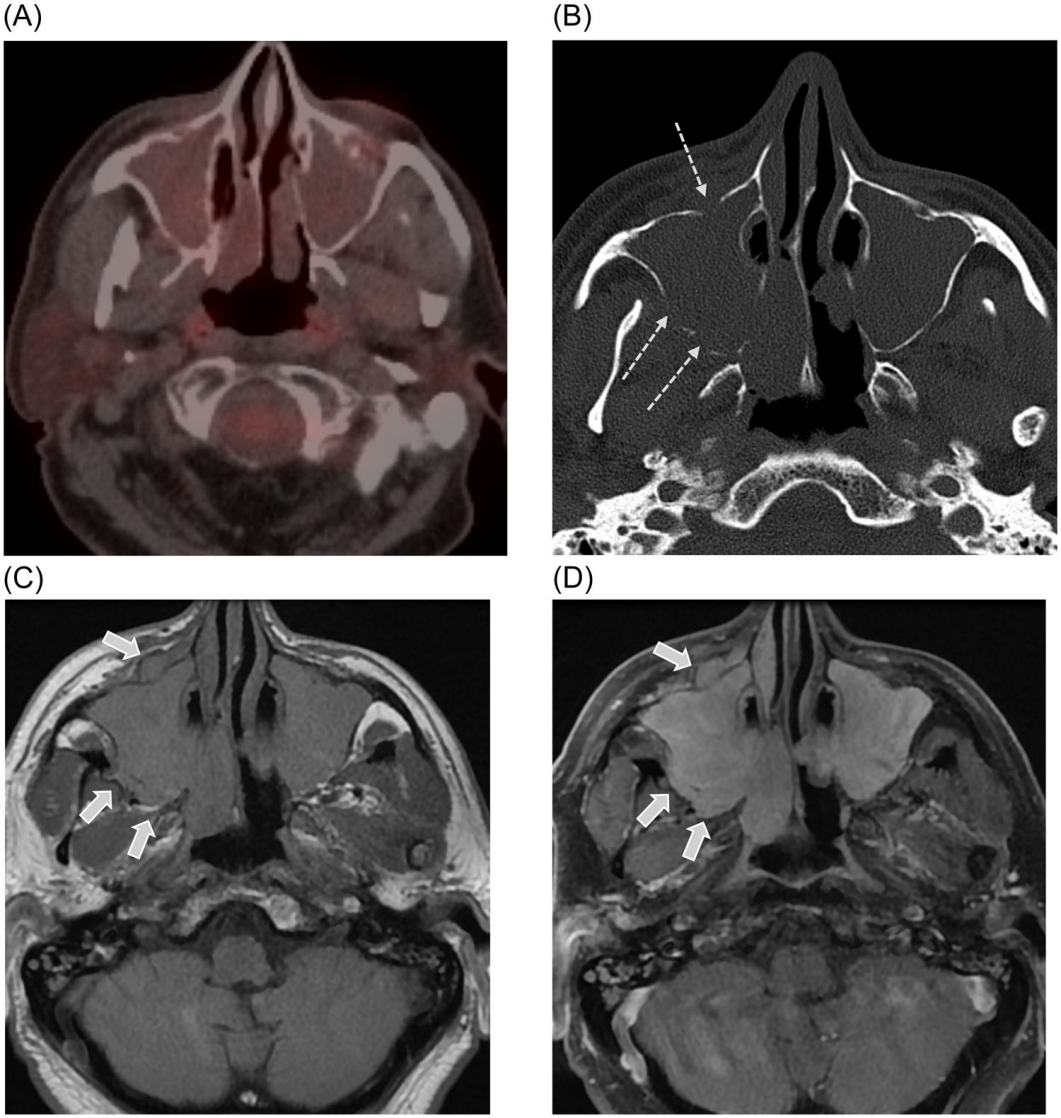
Positron emission tomography/computed tomography (PET/CT), sinus CT, and magnetic resonance imaging (MRI) images in a 74‐year‐old male with a history of multiple myeloma on maintenance chemotherapy for multiple myeloma, presenting with nasal congestion. (A) PET/CT shows diffusely opacified bilateral maxillary sinuses, without significant fluorodeoxyglucose uptake, interpreted as benign. (B) Follow‐up sinus CT 1‐month later shows progressive osseous dehiscence (thin dashed arrows), worrisome for a malignant process. (C, D) Follow‐up MRI precontrast and postcontrast T1 weighted images shows diffuse homogeneous enhancement.
